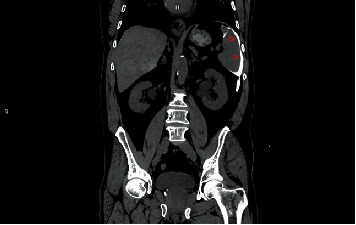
CT–free contrast around the gastric fundus and spleen.
